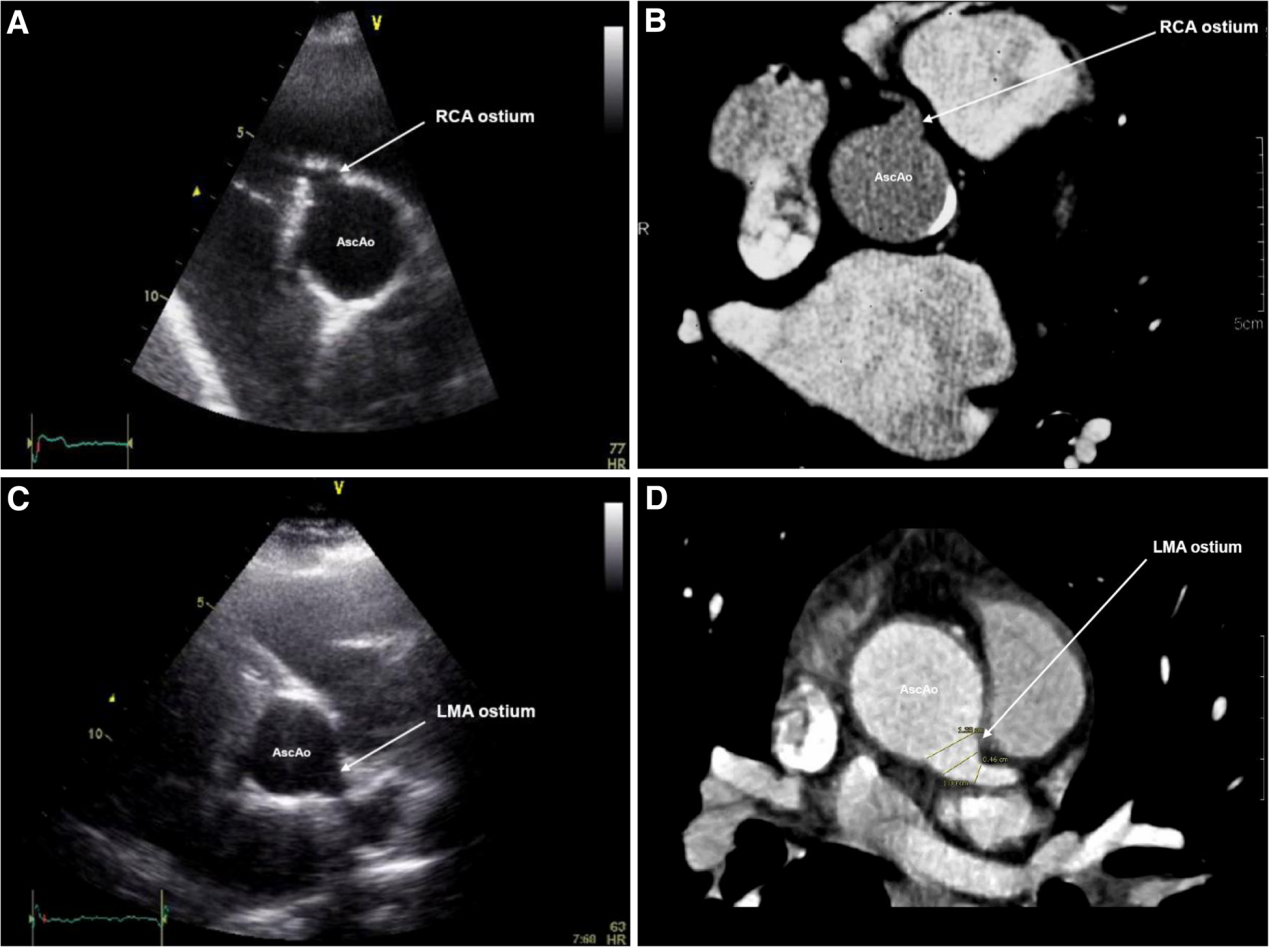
Examples of imaging the coronary artery-aortic graft anastomotic site using transthoracic echocardiogram (TTE) and multi-detector computed tomography (MDCT). A, an off-axis TTE acoustic window derived from the parasternal short-axis view of the right coronary artery (RCA) ostium of patient no.1 at the coronary-aortic graft anastomotic site (patient no.1) and B, visualization of the RCA using MDCT of the same patient (no.1); C, an off-axis TTE acoustic window derived from the parasternal short-axis view of the left main coronary artery (LMA) ostium of patient no.3 at the coronary-aortic graft anastomotic site and D, image of the LMA ostium using MDCT on the same patient (no.3). AscAo, ascending thoracic aorta. All vessel diameters were measured at the coronary ostial-aortic graft junction for both MDCT and TTE imaging techniques.
